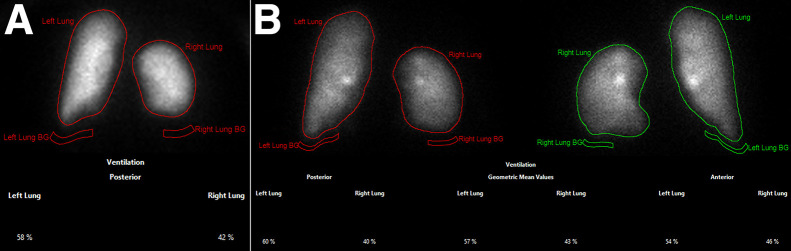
Planar scintigraphy images obtained after inhalation of ^133^Xe in posterior projection (A) and ^99m^Tc-Technegas in posterior and anterior projections (B) in patient with representative images of semiautomated ROIs used for deriving relative ventilation percentages. Measured relative ventilation of left lung was 58% with ^133^Xe, 60% on posterior ^99m^Tc-Technegas image, and 57% with ^99m^Tc-Technegas geometric mean. BG = background.
